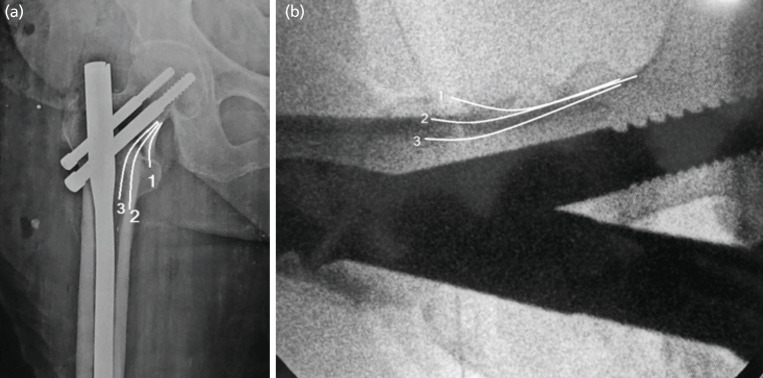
Shows anteromedial cortical support placements, (a) anteroposterior and (b) lateral view of radiographic images, respectively. Line 1 shows positive, line 2 shows neutral or anatomical and line 3 shows negative cortical reduction, respectively.
